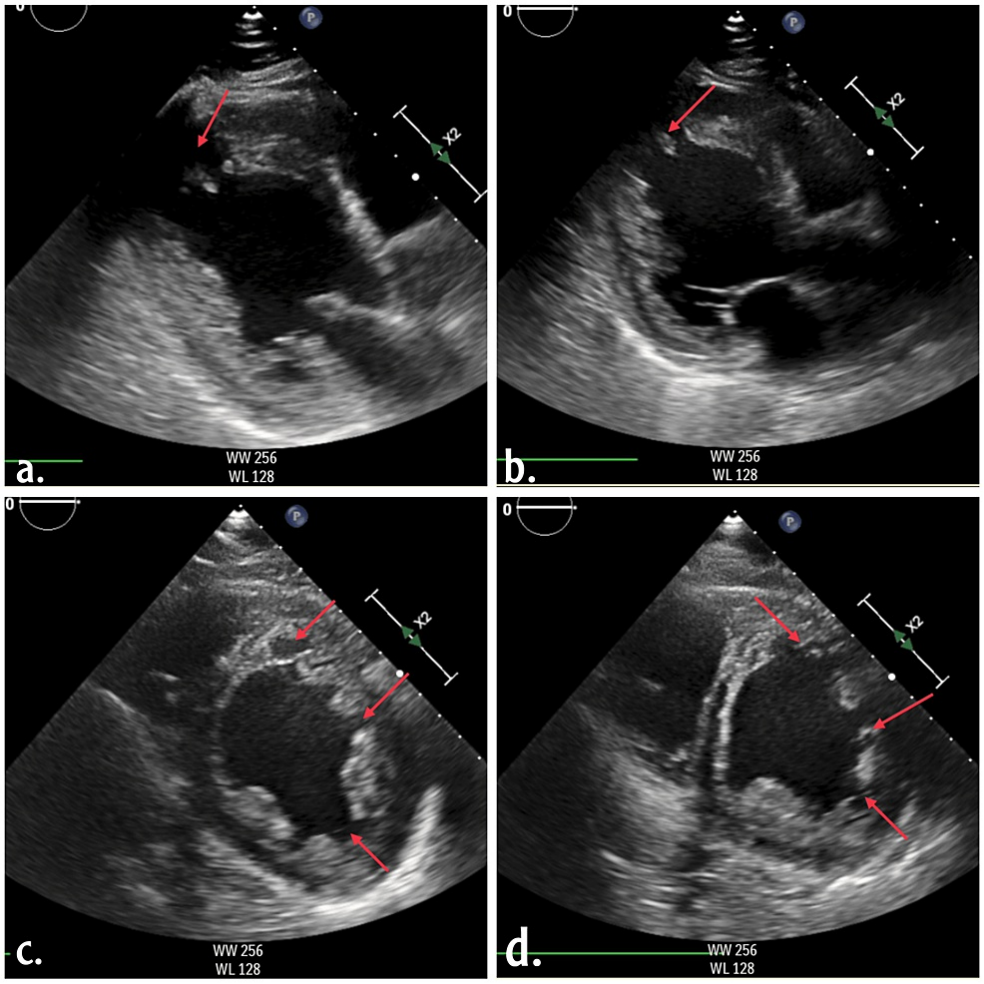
Echocardiogram (a-d) indicates dilated left ventricle internal dimensions, heavy trabeculations, global hypokinesia, ejection fraction was 20-25% by 2D eyeballing, the right side was normal in size with normal right ventricular function, no intracardiac masses or thrombi, normal pericardium with no pericardial effusion.
